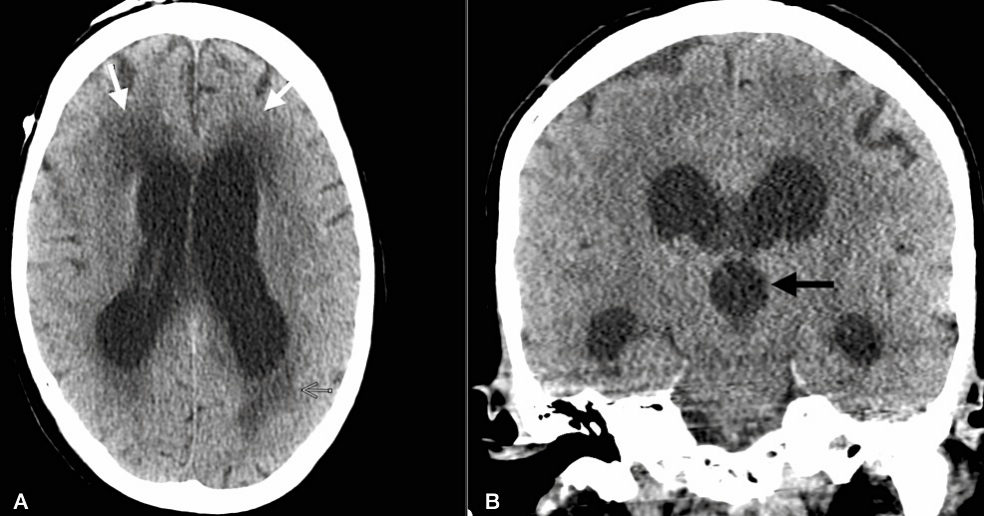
Axial CT image (A) shows marked enlargement of the ventricular system compared to previous CT with increased white matter hypoattenuation adjacent to the ventricular system (straight white arrows), suggestive of worsening hydrocephalus. Coronal CT image (B) shows dilated third ventricle (straight black arrow), which was not seen on previous CT, suggestive of worsening hydrocephalus.
